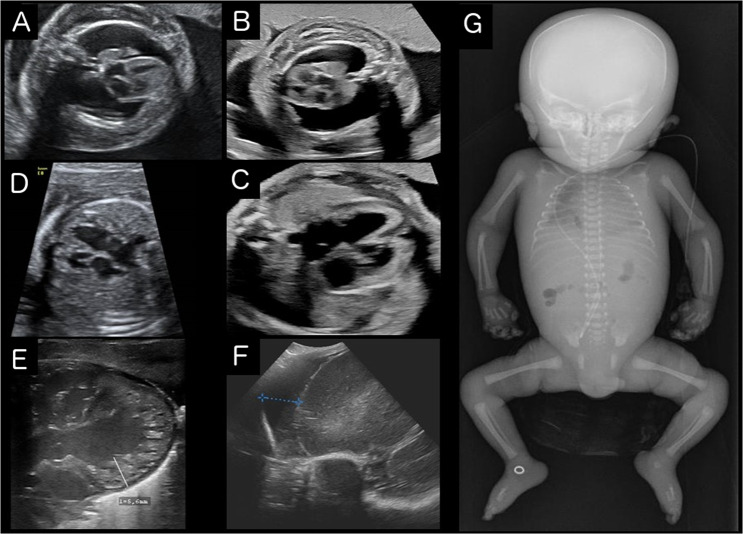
Imaging findings of the affected individuals. Prenatal ultrasound scans at the level of four-chamber view of individuals of family 1 (A: Voluson S8, AB2-7 convex abdominal ultrasound transducer 2–8 MHz; B: Voluson E8, RAB 6-D convex abdominal transducer 2–8 MHz; C, D: Philips EPIQ Elite, V 2–7 convex abdominal ultrasound transducer 2–9 MHz): fetus 1.1 at 28 weeks of gestation (A) and fetus 1.3 at 31 weeks of gestation (B) showing edematous skin and lungs maximally compressed by massive pleural effusions, polyhydramnios. Prenatal myocardial hypertrophy in fetus 1.2 at 25 weeks of gestation (C) and in fetus 1.3 at 28 weeks of gestation (D). Postnatal ultrasound scans of individual 1.2 (Zonare ZS3, Mindray, USA): E spongy left ventricular myocardium (nonstandard plane nonstandard with 20 MHz linear transducer), F frontal hygroma in parasagittal view (blue crosses mark a distance of 1.2 cm, 7,5 MHz curved array transducer). G Postmortem babygram of individual 1.2 (born 31^+2^, deceased on the 4th day of life) showing thickened soft tissue and pleural effusion on the right side, normal skeletal findings (color figure online).
